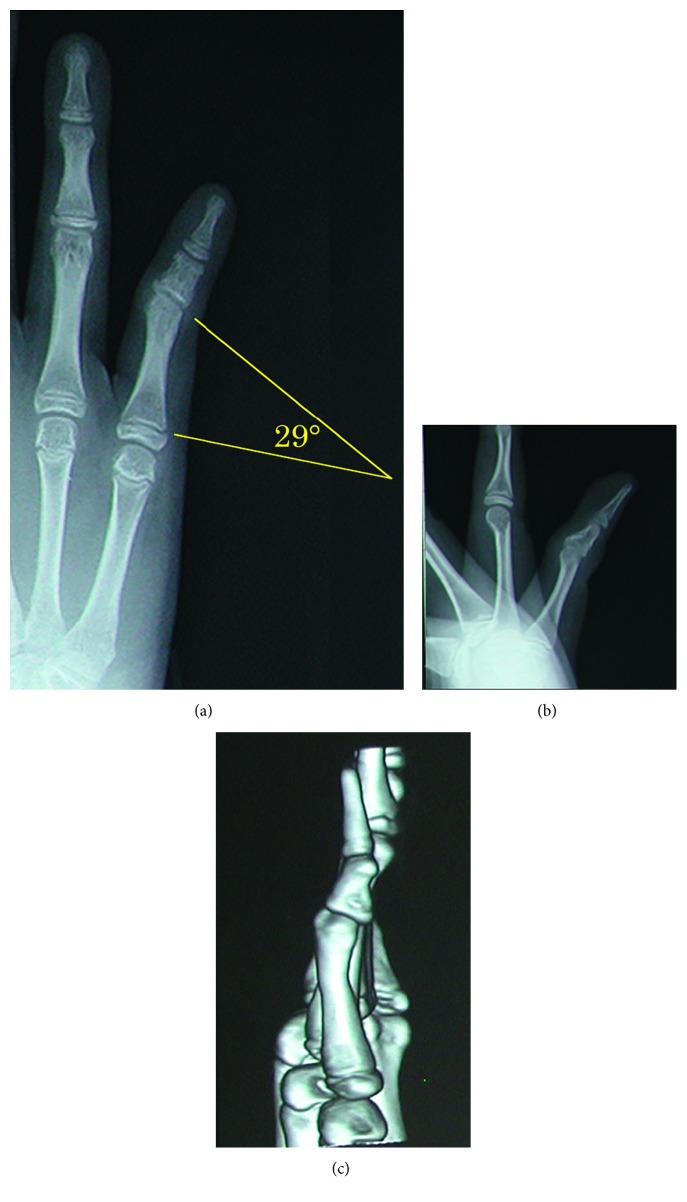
Preoperative imaging in Case 1. (a) Plain radiographic anteroposterior view of the right little finger. The distal articular surface of the proximal phalanx of the little finger was tilted in the ulnar direction. (b) Plain radiographic lateral view of the right little finger. (c) Three-dimensional computed tomography of the proximal phalanx of the right little finger.
